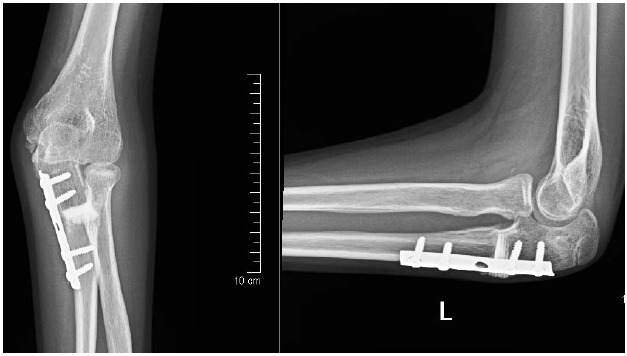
Radiograph one year after open reduction of the radial head with angulating-distraction ulnar osteotomy, showing well reduced radial head and incorporation of interpositional phosphocalcic ceramic wegde graft.
